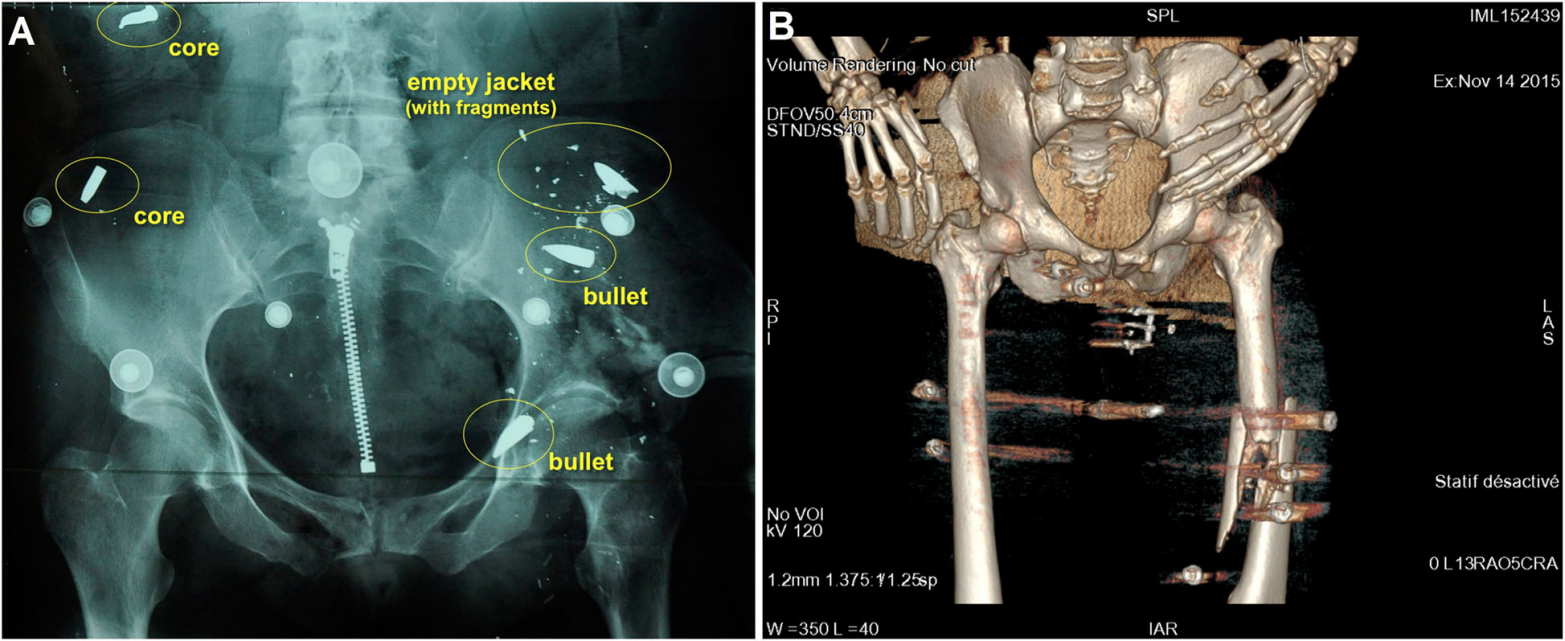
(A) Representative standard pre-autopsy X-ray image allowing identification of various kinds of intracorporeal ballistic material. (B) Representative 3D-reconstructed pre-autopsy CT imaging lacking precision for identifying the various kinds of intracorporeal ballistic material.
